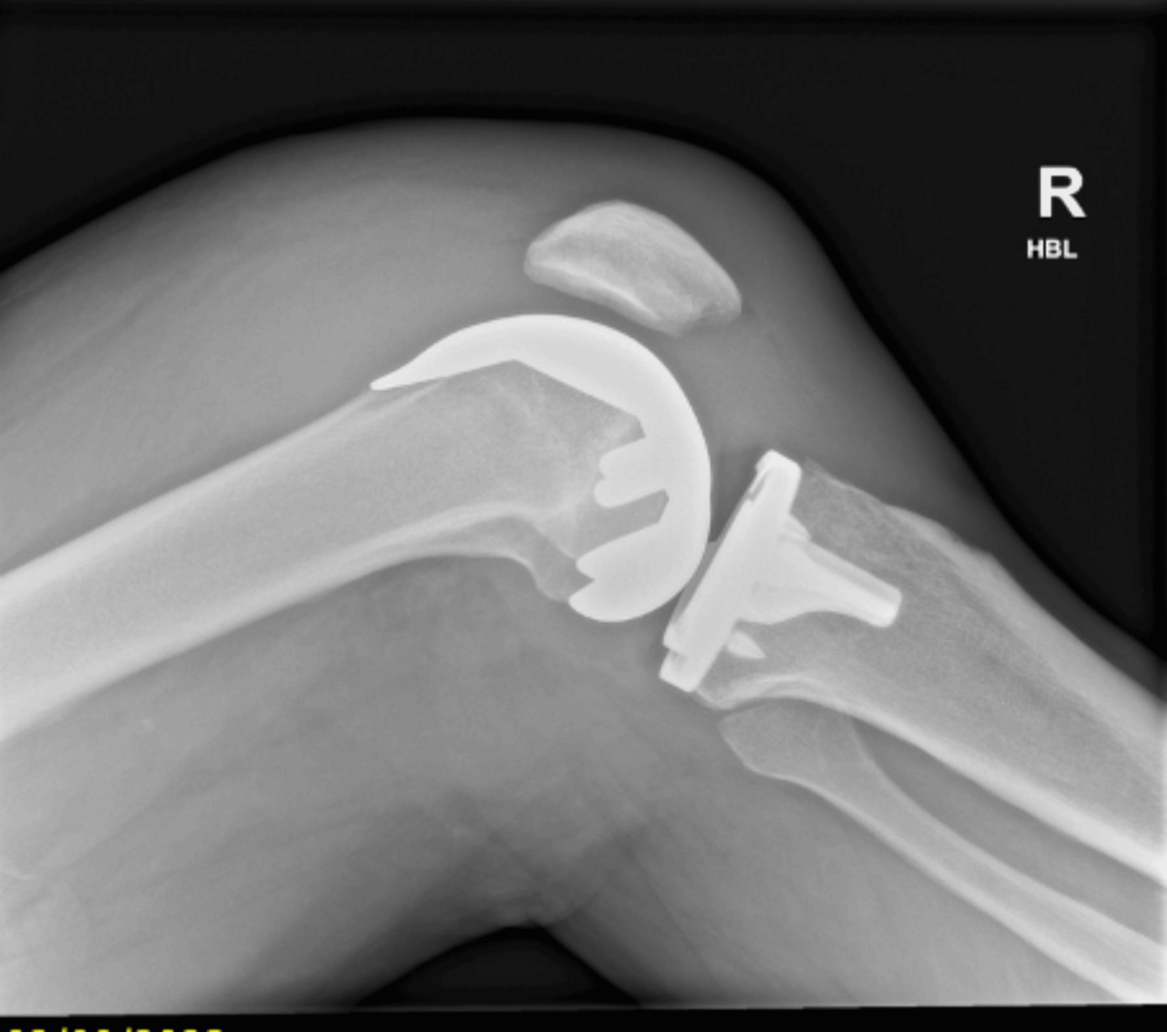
Postoperative lateral radiograph of the knee showing total knee replacement in place and gross joint effusion in form of loss of supra and infrapatellar fat pad.
